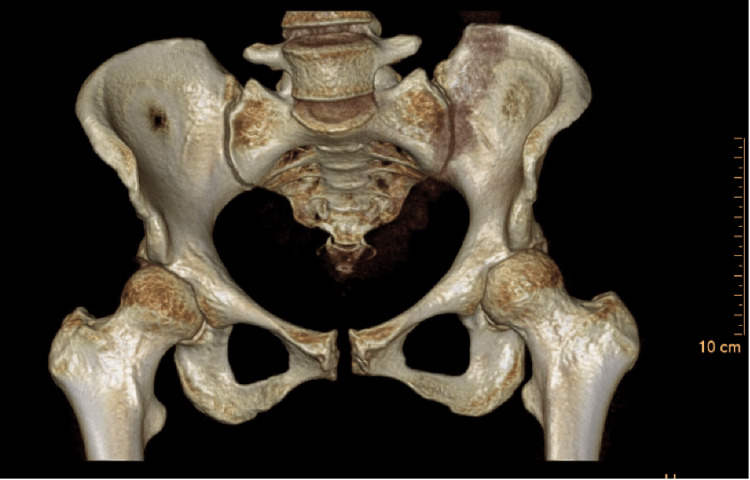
Computed tomography (CT) scan 3D reconstruction of the patient's pelvis demonstrates pubic symphyseal widening
